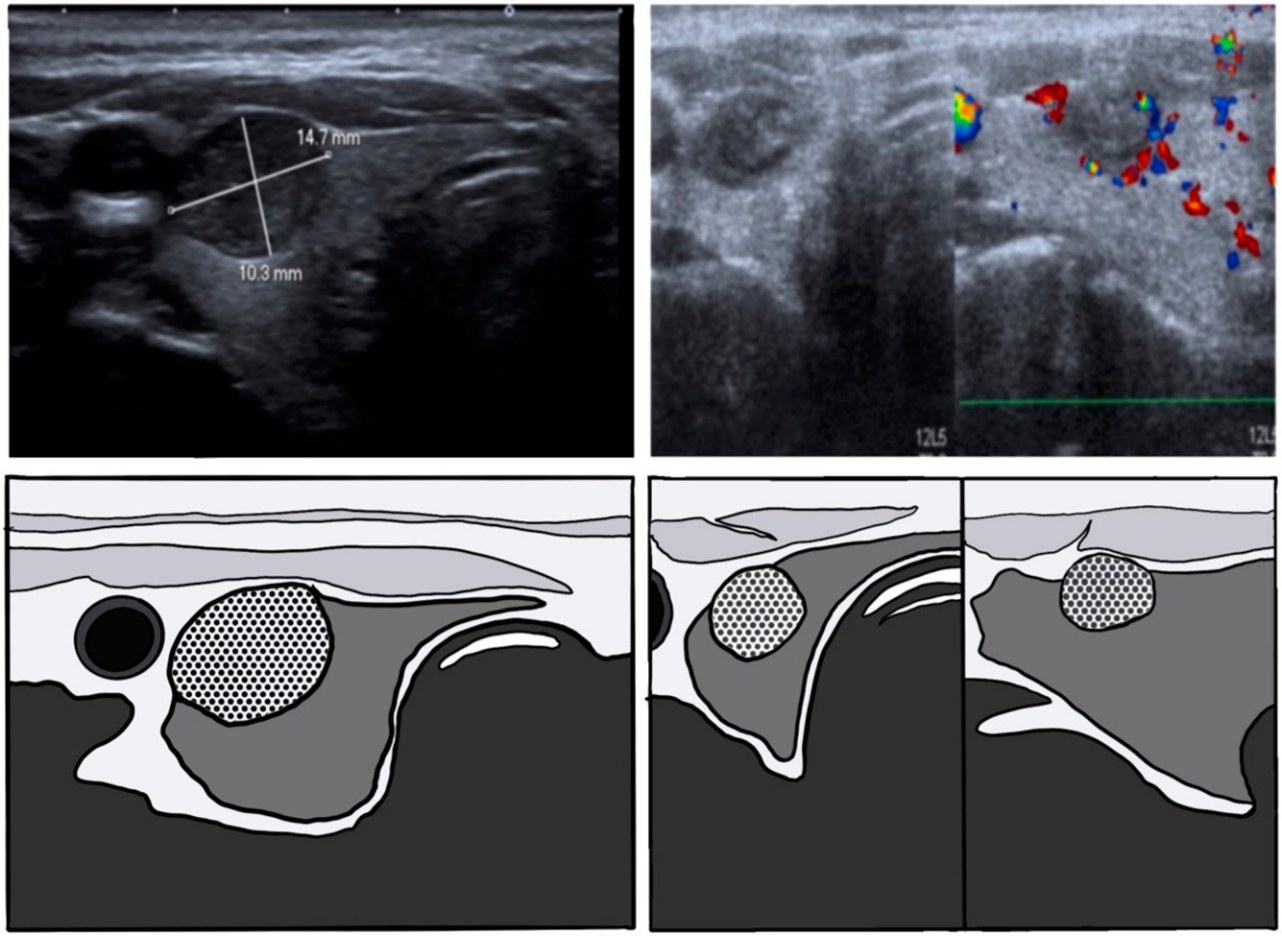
Usual reduction in the size of the nodule and alteration of the echogenicity in the ultrasound control at 12 months after ablation.
